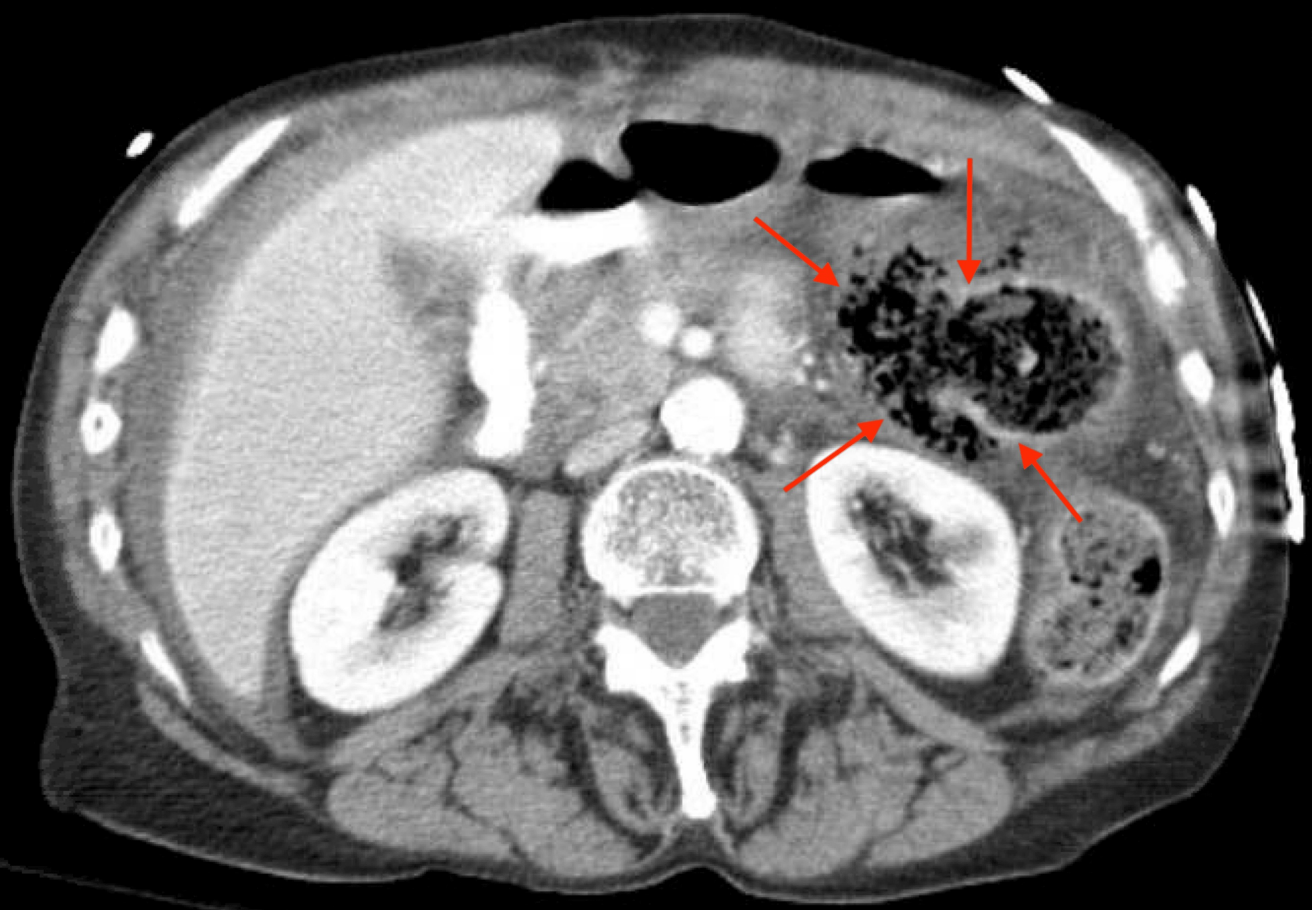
Axial computed tomography of abdomen and pelvis with contrast, showing a perforated viscus in the distal colon (red arrows) with intraperitoneal free air
